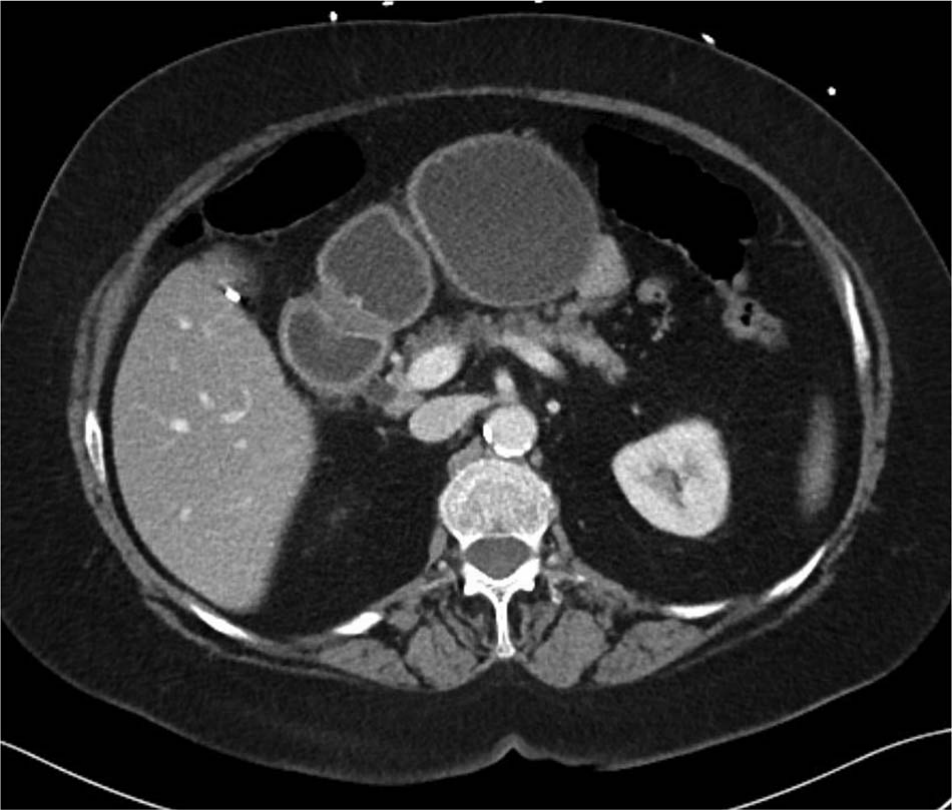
CT scan A/P of the abdomen and pelvis with IV contrast on presentation showing moderately dilated gastric remnant with thickening of the pylorus and proximal duodenum.
